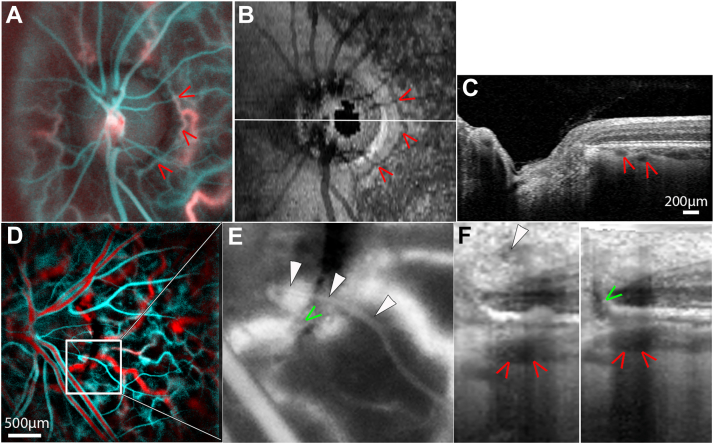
A–C, Paraoptic arteries circling the optic nerve head. A, Laser Doppler holography (LDH) image of a circular paraoptic artery, possibly the Zinn–Haller circle; B, en face OCT; C, transverse OCT along the line in (B) showing the subretinal pigment epithelium location of paraoptic arteries. D–F, Emergence of a cilioretinal artery. D, The LDH image; E, magnification showing the cilioretinal artery (white arrowhead). The green arrowhead shows the connection between the cilioretinal artery and the paraoptic artery on the LDH image and on the transverse OCT (F).
